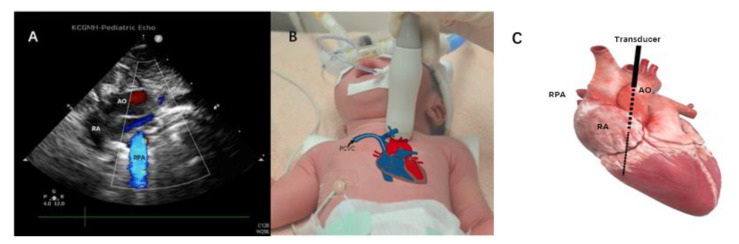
The probe was placed on the baby’s left parasternum, at the upper border between nipples. The black arrow indicates the percutaneous central venous catheter (PCVC) in situ. (A) Aorta in the left parasternal longitudinal view, (B) location of the transducer, and (C) the heart anatomy with the probe position indicates a black solid line and black dash line for the plane of ultrasound. AO, aorta; RPA, right pulmonary artery; RA, right atrium.
